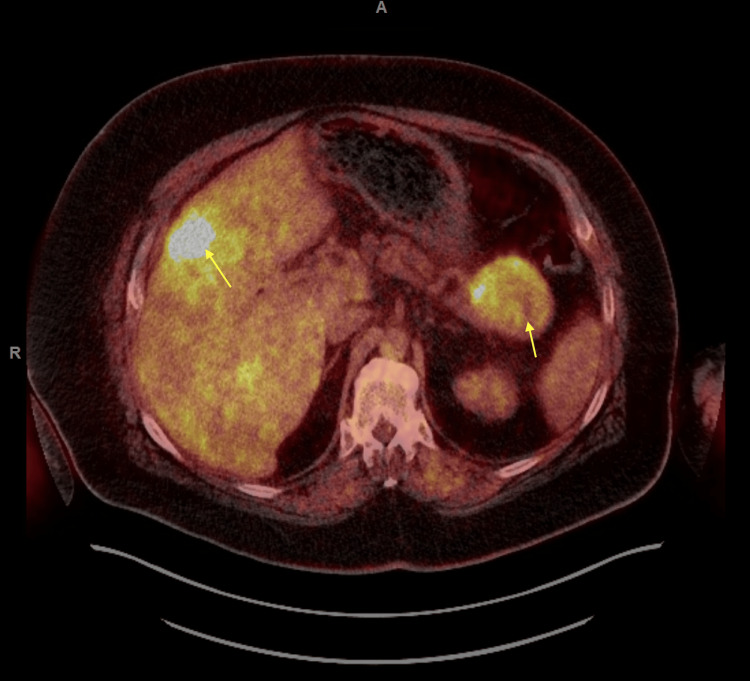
FDG PET/CT scan of the whole body showing hypermetabolic pancreatic tail mass which measures up to 6.5 cm and multifocal liver hypermetabolic metastases. FDG: fluorodeoxyglucose; PET: positron emission tomography; CT: computed tomography
